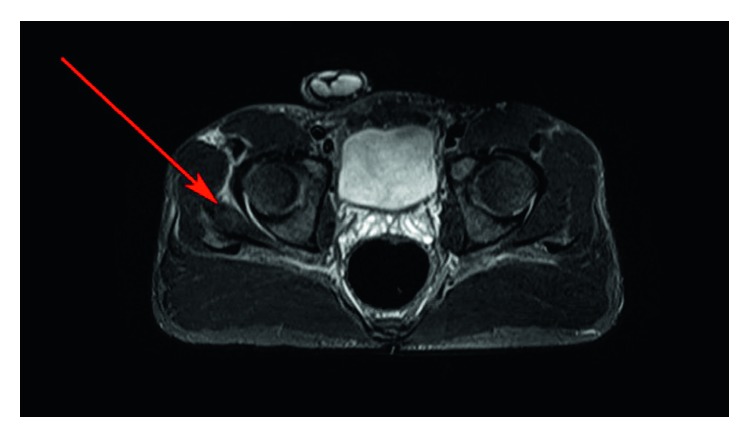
MRI (red arrow) shows moderate intramuscular edema at the ventral aspect of the right gluteus minimus muscle with mild muscle enhancement.
